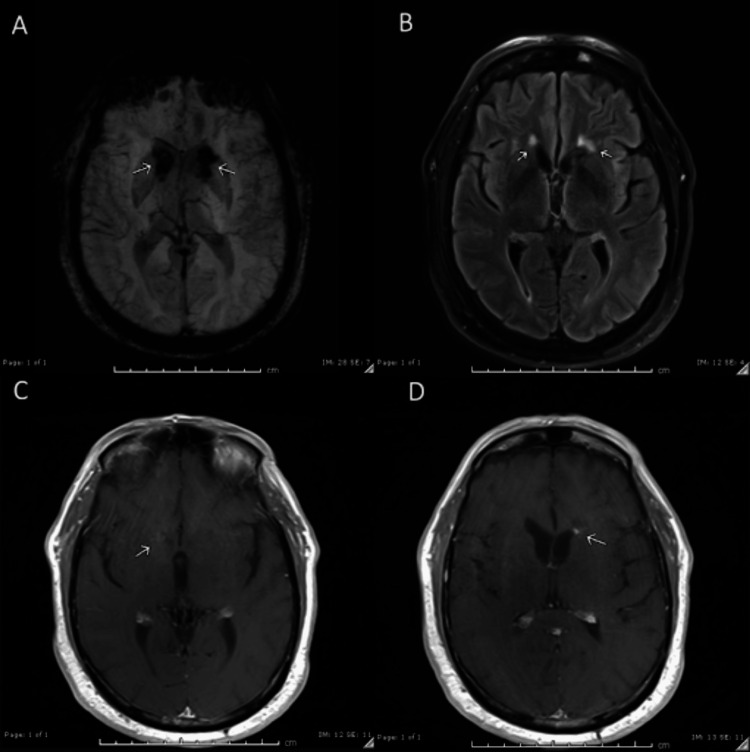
Repeat MRI 250 days after the original MRI (A) SWI: Persistent, possibly increased susceptibility artifacts in the caudate heads compatible with hemosiderin deposition/underlying hemorrhage, unclear whether related to differences in the imaging techniques. (B) FLAIR: Continued interval decreased size of the signal abnormalities involving the caudate nuclei. (C-D) T1 with contrast enhancement: Interval resolution of the enhancement in the right caudate head and significantly decreased enhancement in the left caudate head. MRI: magnetic resonance imaging; SWI: susceptibility-weighted imaging; FLAIR: fluid-attenuated inversion recovery
